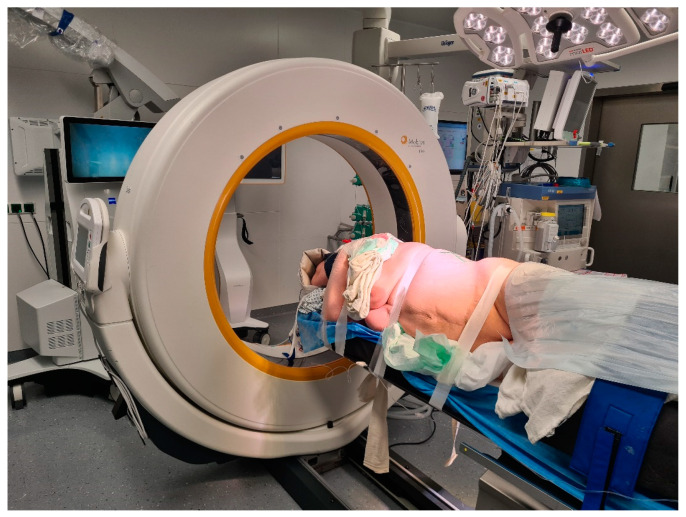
Lateral decubitus position of the patient for a left lateral transthoracic transpleural approach (patient Number 6).
